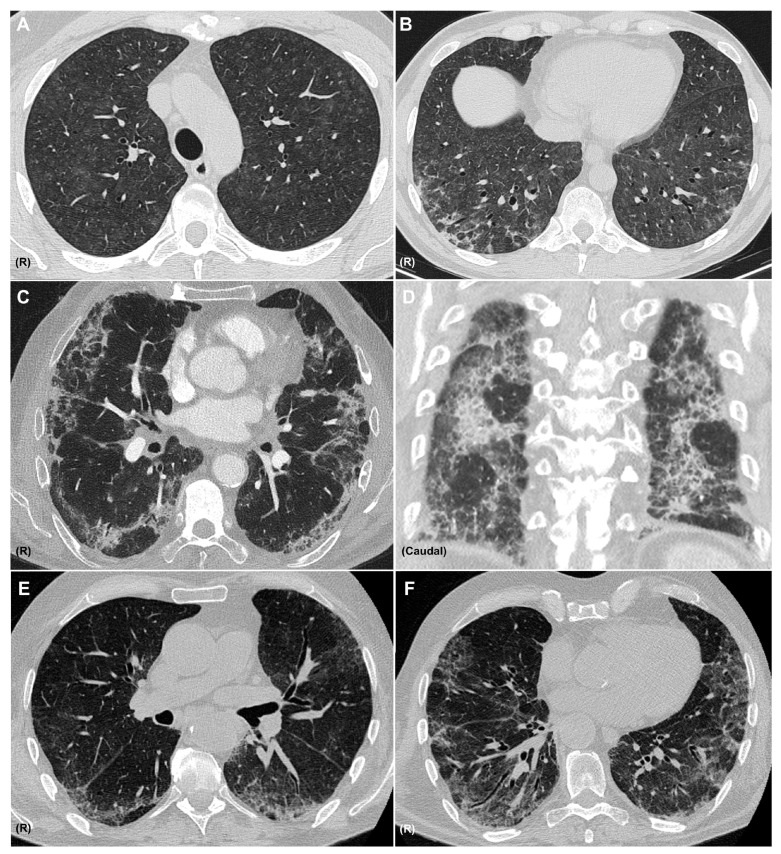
CT Patterns of Immune-Related Pneumonitis. Axial CT images showing a combined pattern, with non-fibrotic hypersensitivity pneumonitis characterized by diffuse centrilobular ground-glass nodules (A) and perilobular consolidative foci consistent with organizing pneumonia (B), in a patient with melanoma treated with nivolumab and ipilimumab. Axial (C) and coronal (D) CT images showing a combined nonspecific interstitial pneumonia (NSIP) pattern with ground-glass opacities, reticulation, and areas of subpleural sparing, along with consolidations consistent with organizing pneumonia, in a patient with pulmonary adenocarcinoma receiving pembrolizumab. Axial CT images (E,F) showing an NSIP pattern with ground-glass opacities, areas of subpleural sparing, reticulation, and traction bronchiectasis in a patient with clear cell renal carcinoma treated with nivolumab. R: right side. All CT images are fully anonymized and contain no identifiable patient information; therefore, no individual consent was necessary.
